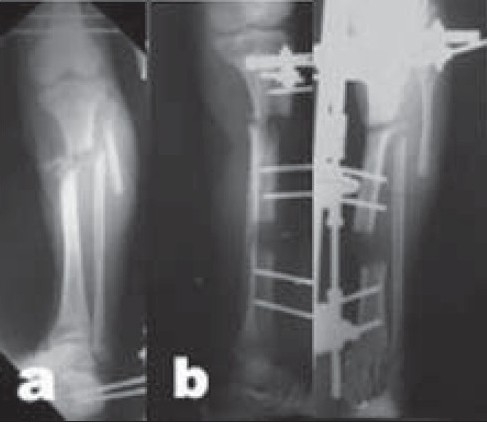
26-year-old male patient who sustained Type IIIB open fracture of the tibia. (a) Radiograph of the leg (anteroposterior view) after being treated elsewhere by gastrocnemius muscle flap, antibiotic beads insertion, shows spanning external fixator. (b) The patient was then referred after application of a monolateral fixator for bone transport. Radiograph of the same leg (lateral and anteroposterior view) shows deviation of the transported segment and distraction at the nonunion site.
